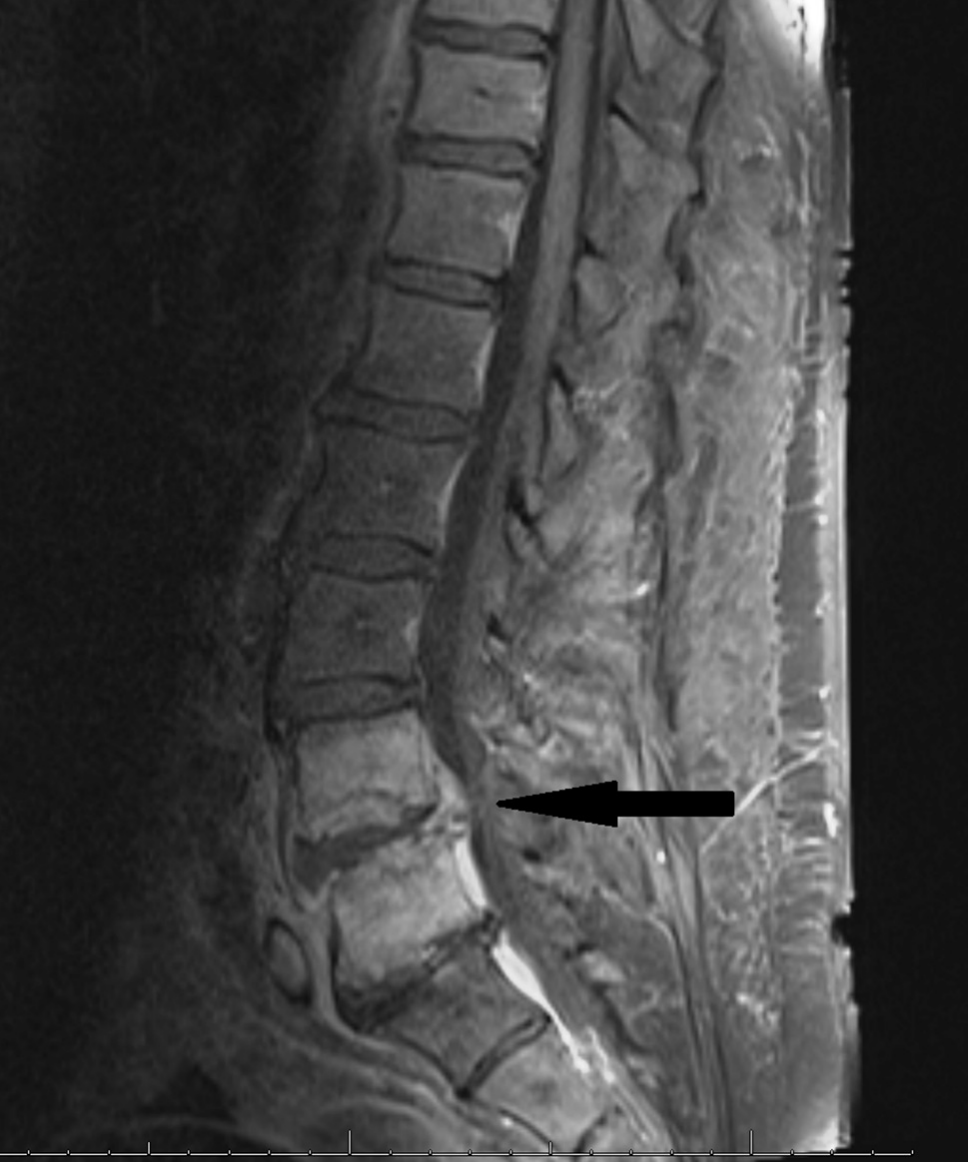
Lumbar spine MRI (T1 sequence with gadolinium enhancement). Arrow indicates L4–5 discitis/osteomyelitis with epidural extension.
